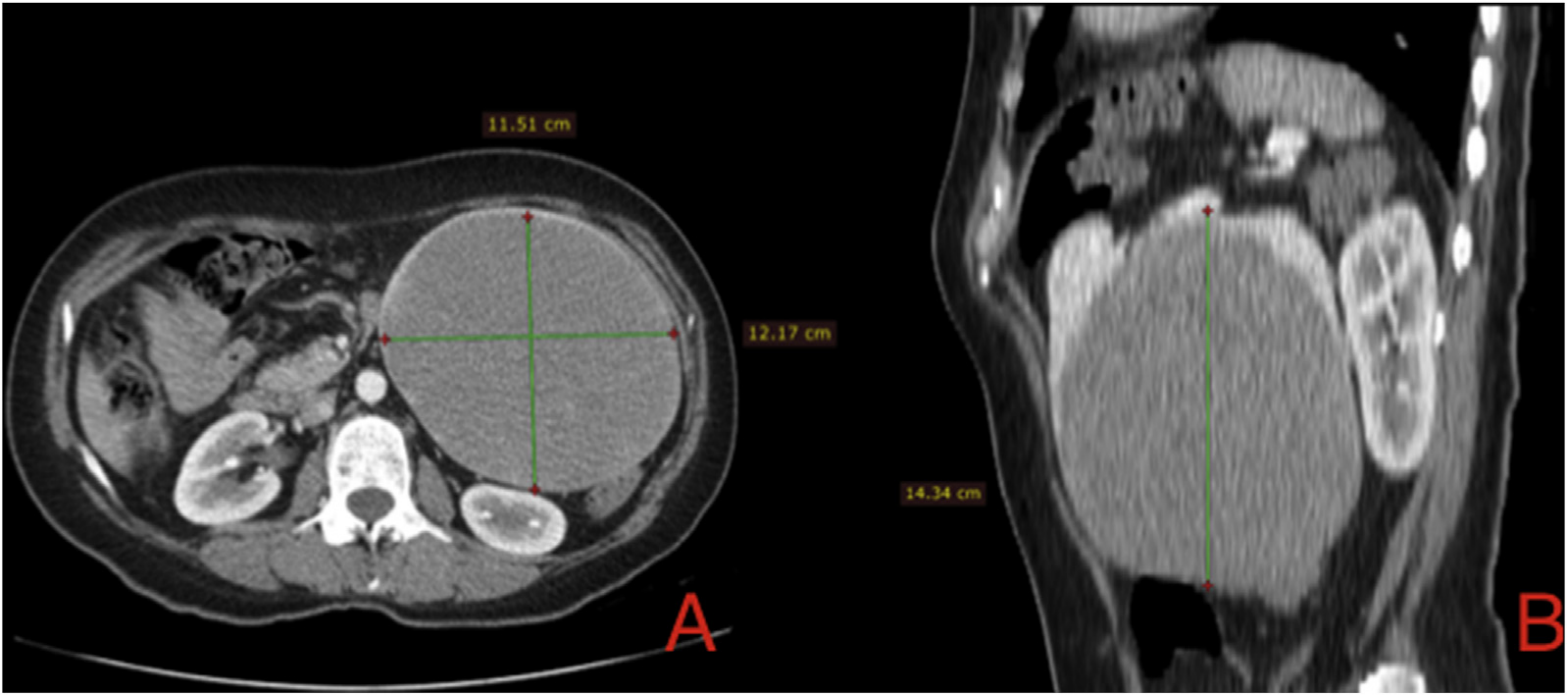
Abdominal CT A) Axial view with a 11.5 × 12.1 cm spleen tumor with heterogeneous enhanced on the arterial phase. B) Sagittal view, with the greatest dimension of the tumor of 14.3 cm.
